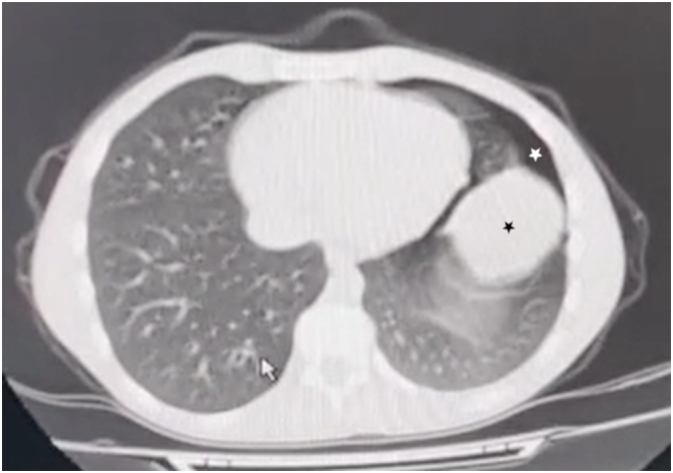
Chest CT scan showing a hydatid cyst lesion (Black start), and a pneumothorax (White star).
